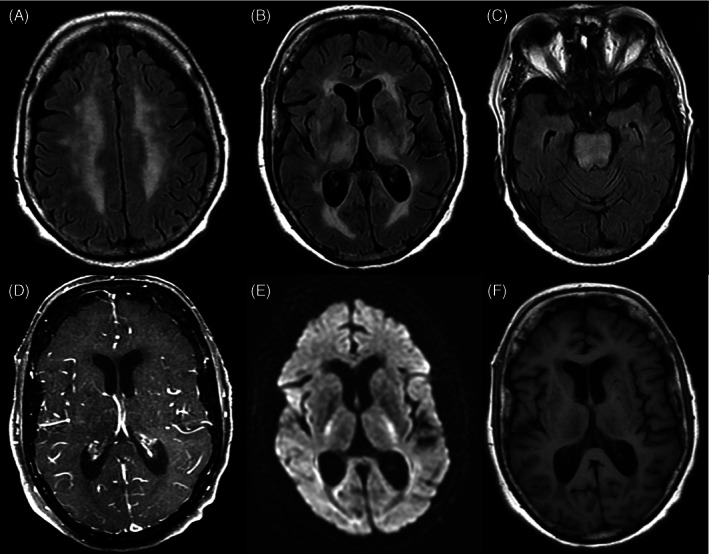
Multiplanar brain MR images of 77‐years old female on Lenvatinib for treatment of metastatic thyroid cancer, who presented with left arm weakness. Upper panel of Fluid‐attenuated inversion recovery (FLAIR) sequence images show hyperintense signal in the centrum semiovale and corona radiata (A), periventricular white matter (B), and brainstem (C). There was no significant post‐contrast enhancement (D), restriction on diffusion weighted images (E) or associated T1 abnormality (F). The T2 hyperintensity along the in the brain‐stem extending along the basal ganglia into the corona‐radiata and centrum semiovale with the given clinical history and treatment details were diagnostic of atypical PRES, likely Lenvatinib induced
